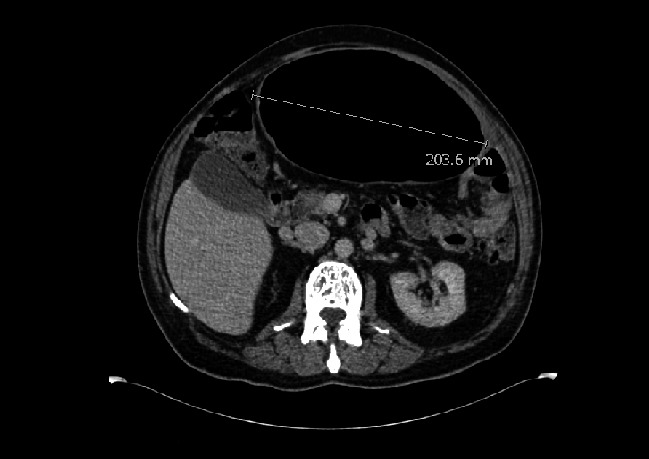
CT showing a 20.3 cm sigmoid mass in keeping with giant diverticulum.
